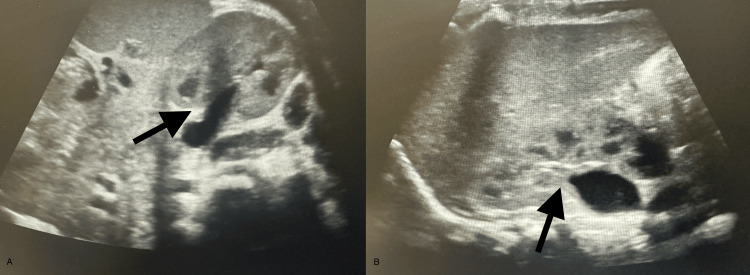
Ultrasound demonstrating bilateral grade 1 hydronephrosis in a seven-week-old infant with pyelonephritis
